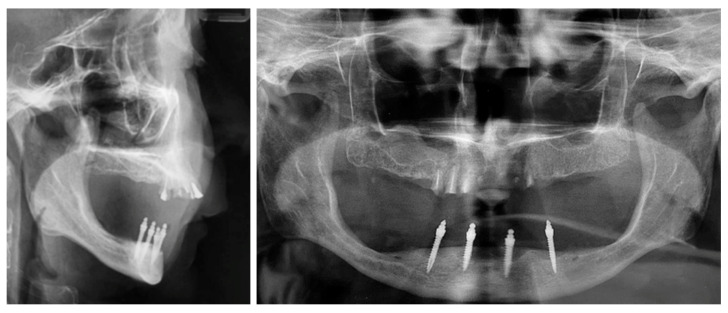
The orthopantomographic and cephalometric radiograph, at 14 years follow-up.
